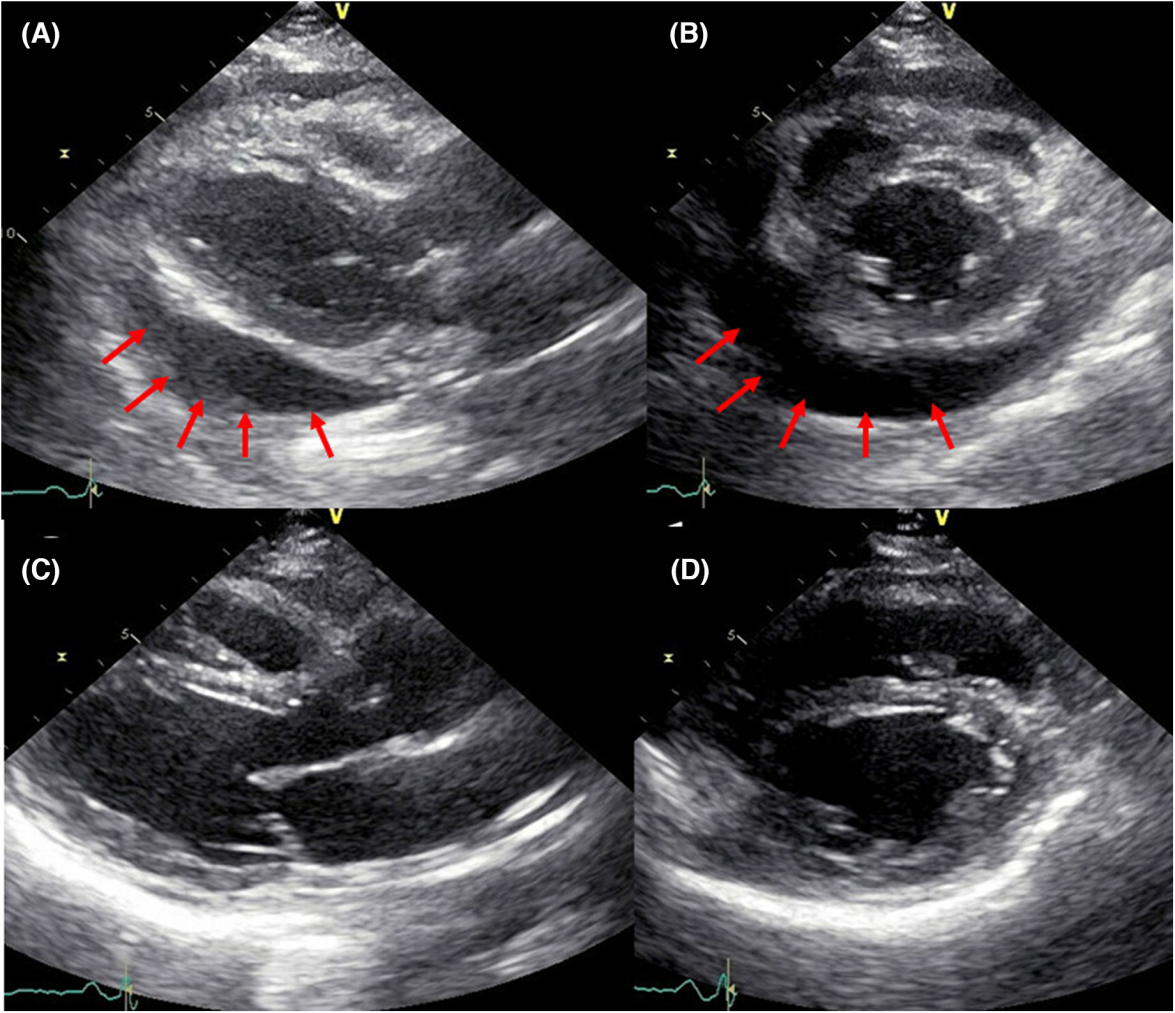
Echocardiography at the initial visit revealed pericardial effusion. (A) Parasternal long axis. (B) Parasternal short axis. Red arrows point to pericardial effusion. Echocardiography after pericardial drainage. Pericardial effusion remained low without excessive reaccumulation. (C) Parasternal long axis. (D) Parasternal short axis.
